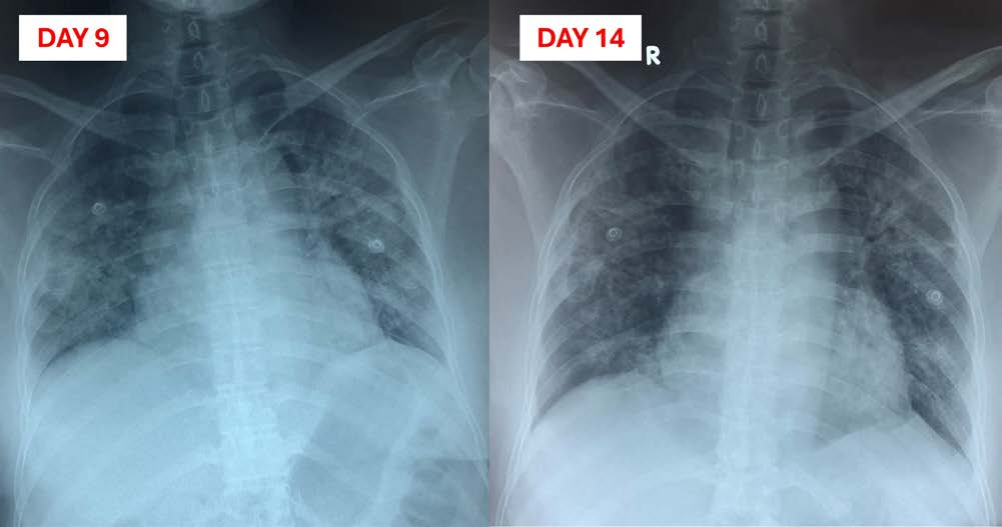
Chest X-ray from day 9 to day 14 postadmission shows significant reductions in lung consolidations compared to Figure 1.
